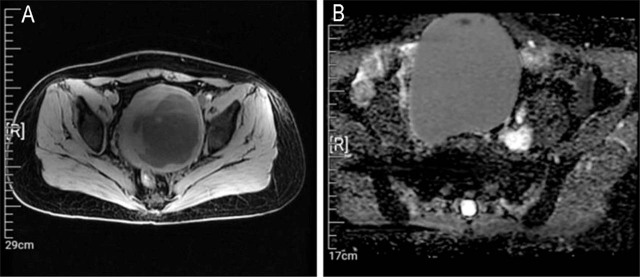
Magnetic resonance imaging and macroscopic findings. (A) T2-weighted image and (B) diffusion-weighted image depicting a high signal intensity in the uterine fibroid.
